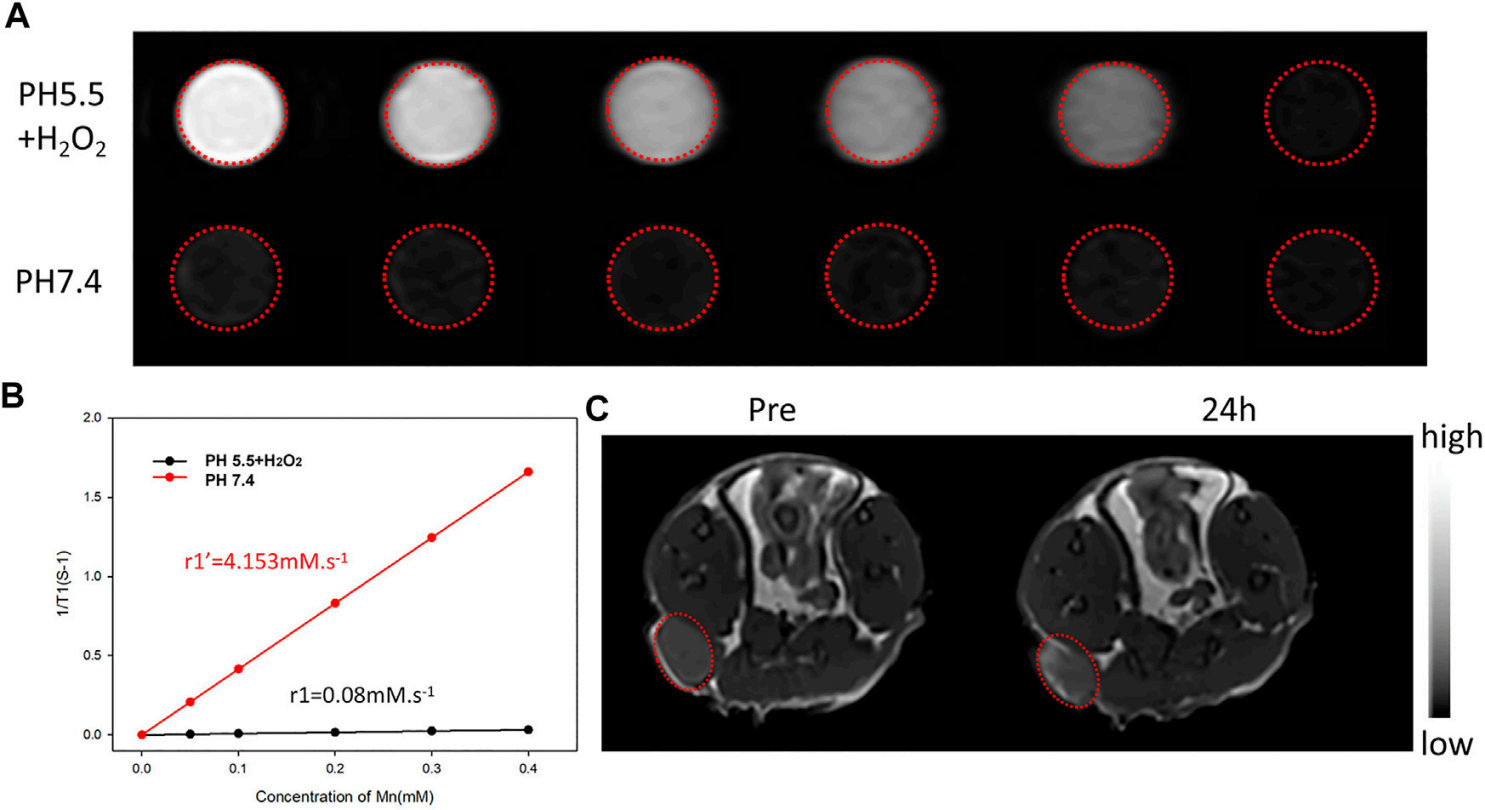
(A) T1-weighted MR images of IR780/PLGA@MnO_2_ NPs suspensions at different Mn concentrations and pH values with H_2_O_2_. (B) The longitudinal (r1) relaxation rate *vs.* the concentration of the IR780/PLGA@MnO_2_ NPs (in terms of Mn). (C)
